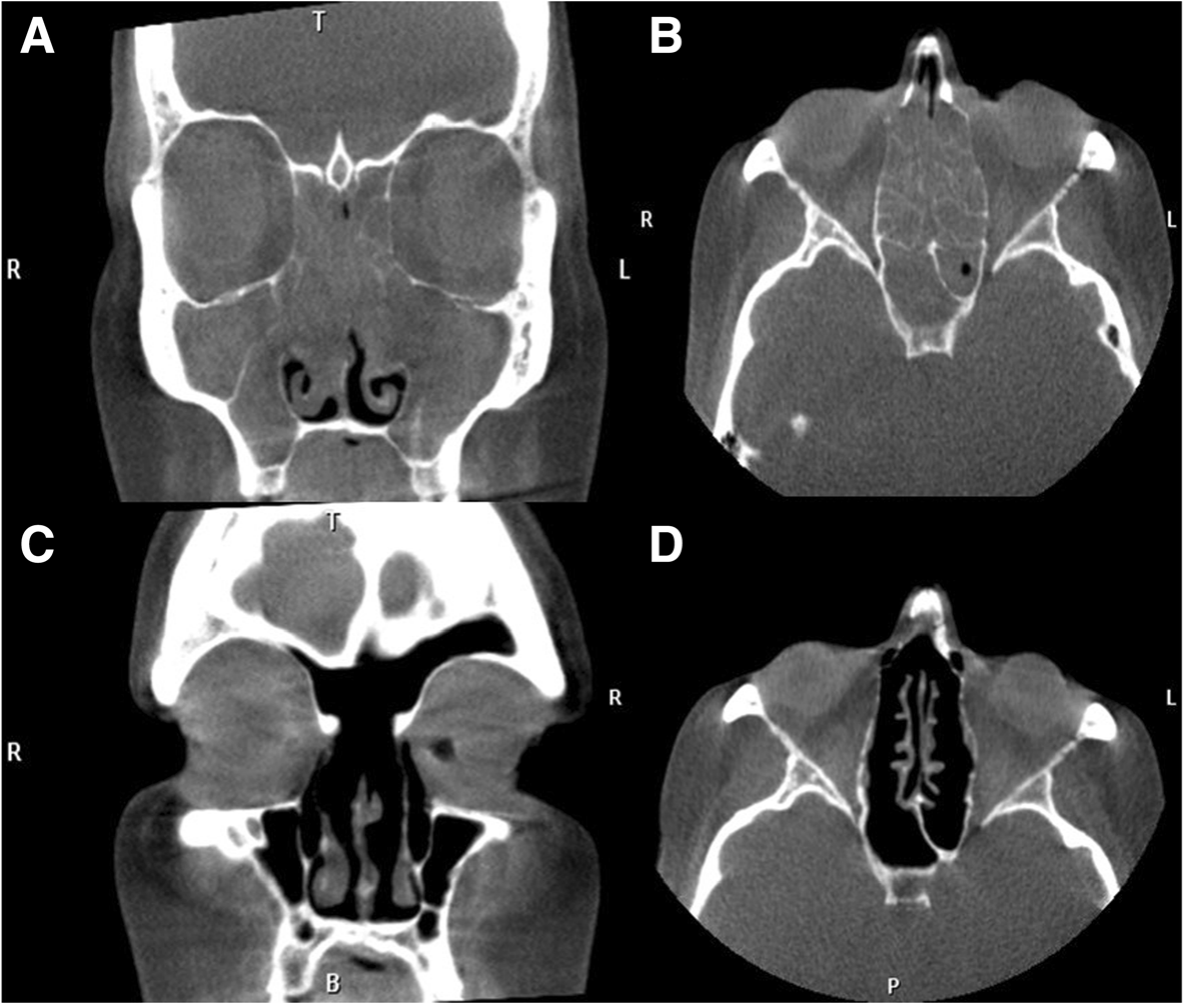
a,b: Pre-operative CT images view of patient suffering from eCRS; c,d: Post-operative CT images of patient compliant on corticosteroid irrigations
